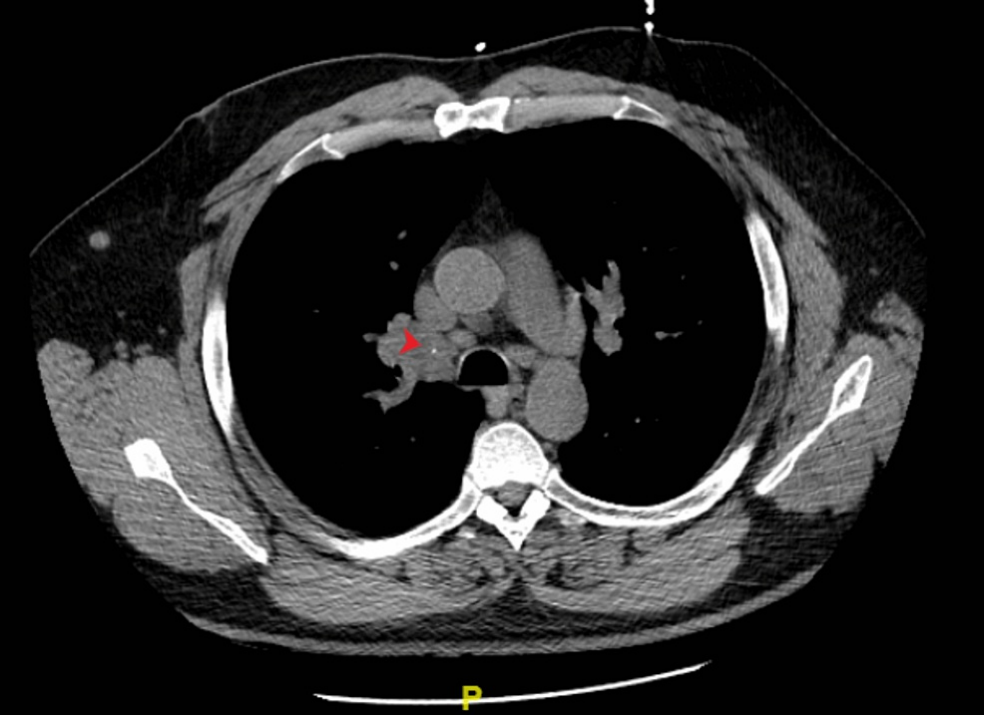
Axial view of chest CT without contrast showing calcified right hilar adenopathy (arrow)
